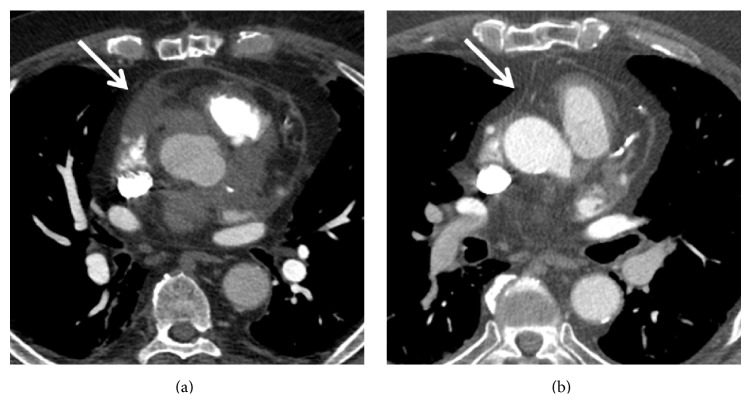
Contrast-enhanced computed tomography, subsequent images through heart in the patient with GPA show soft tissue attenuation around the pericardium (a). Resolution of pericardial thickening after the immunotherapy (b).
